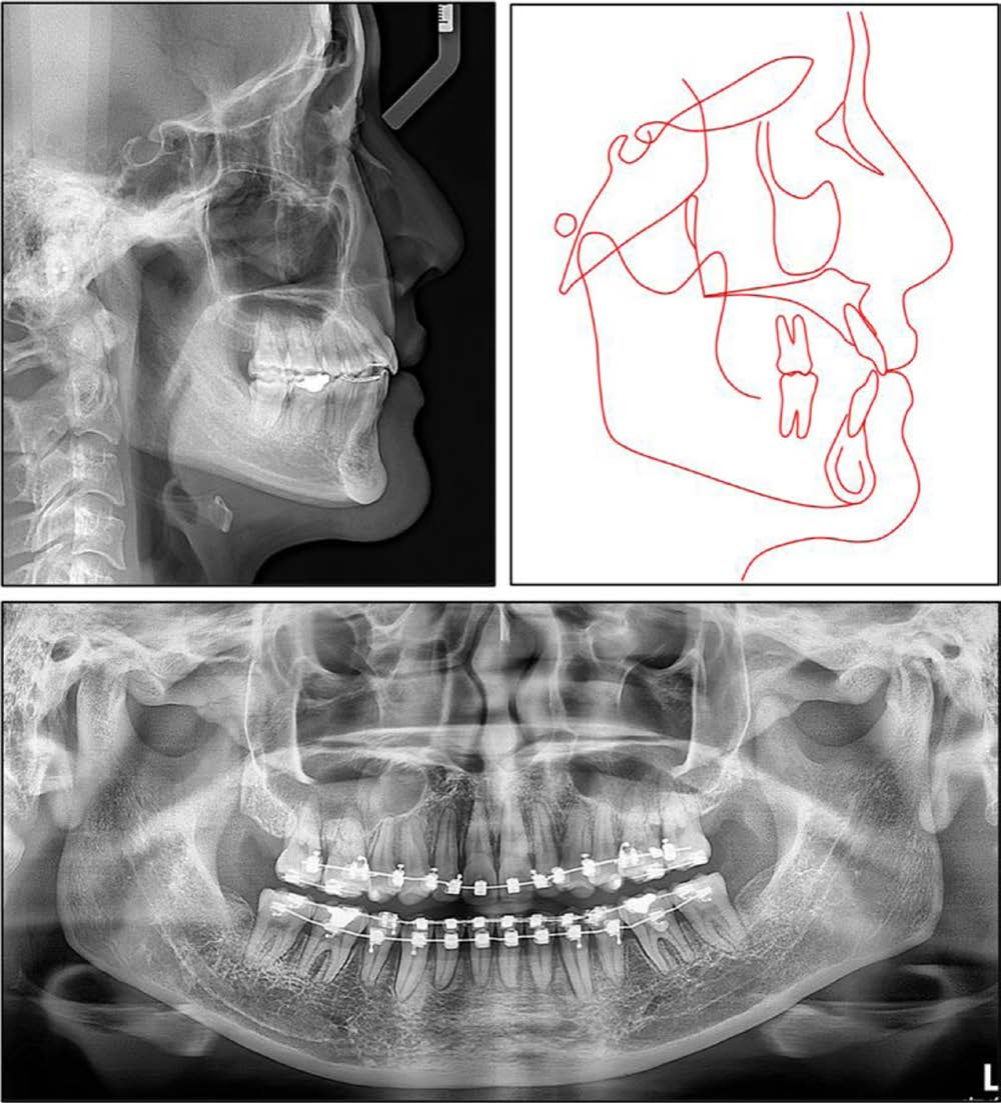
Posttreatment lateral cephalogram, cephalometric tracing, and panoramic radiograph.
